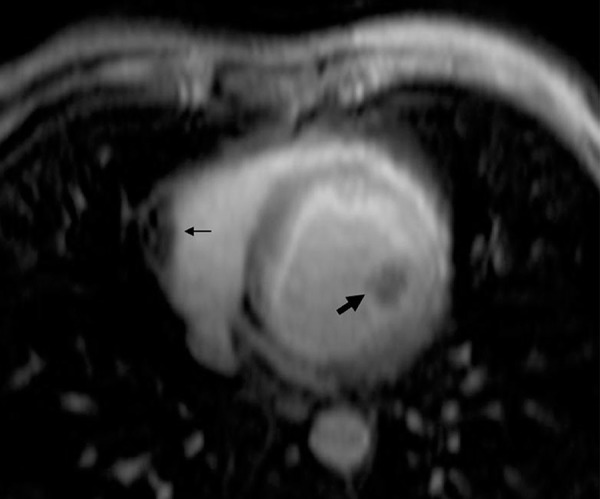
Cardiac Magnetic Resonance Imaging Showed Cystic Lesions, in the Left Ventricular Posterolateral Wall Protruding into the Lumen (Thick Arrow) and Right Ventricular Free Wall Expanding to Apical Segment. (Thin Arrow)
